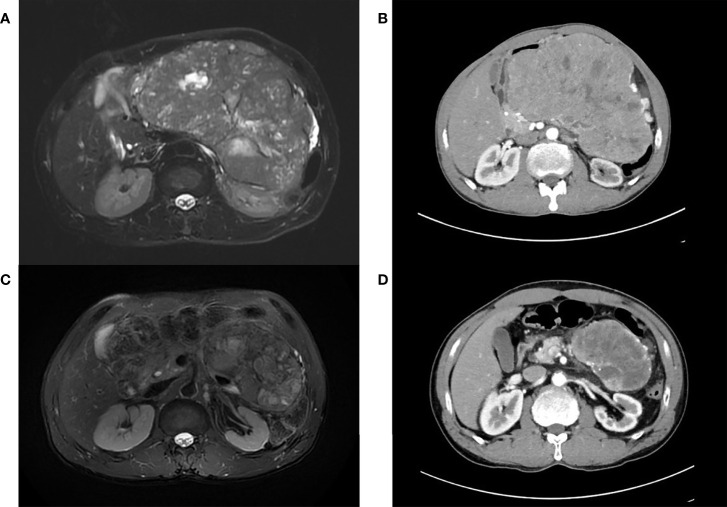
(A) Pretreatment magnetic resonance imaging (MRI) image depicting a multilobulated tumor with the size of 16.1 cm × 14.9 cm × 14.5 cm in the left upper abdomen. (B) Tumor progression up to the greatest diameter of 18 cm in the axial view of computerized tomography (CT) scans after treatment of Lanreotide. (C) Prominent tumor shrinkage after concurrent chemoradiation. (D) At least 50% reduction of tumor axial perpendicular diameters in preoperative CT.
